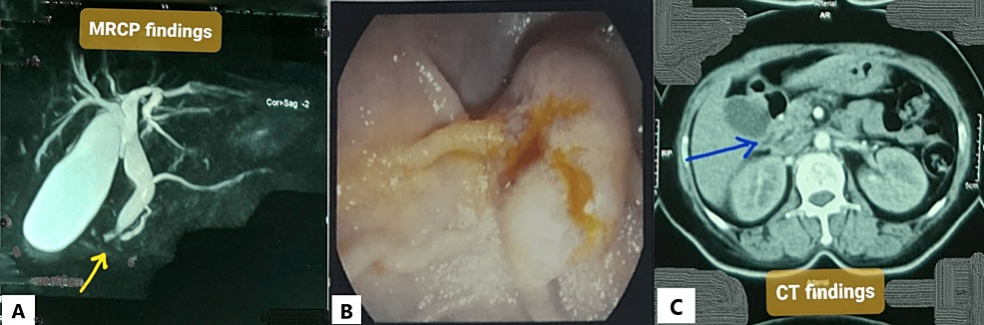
(A) MRCP findings suggestive of dilated CBD and MPD also known as the double-duct sign (yellow arrow). (B) UGI endoscopy showing the bulged-out ampulla. (C) Axial view of the CECT of the chest and upper abdomen showing an ill-defined, minimally enhancing soft tissue lesion in the periampullary area with dilated MPD (blue arrow). MRCP: magnetic resonance cholangiopancreatography; CBD: common bile duct; MPD: main pancreatic duct; UGI: upper gastrointestinal; CECT: contrast-enhanced computed tomography
